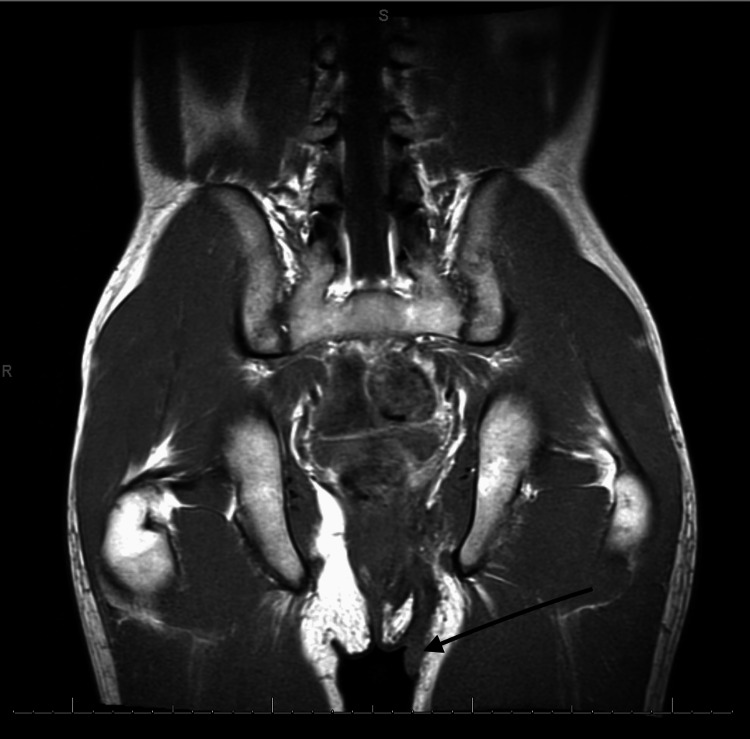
MRI of the pelvis shows a fistulous tract extending from the left pubococcygeal muscle into the left upper thigh, suggesting an abscess (arrow).
